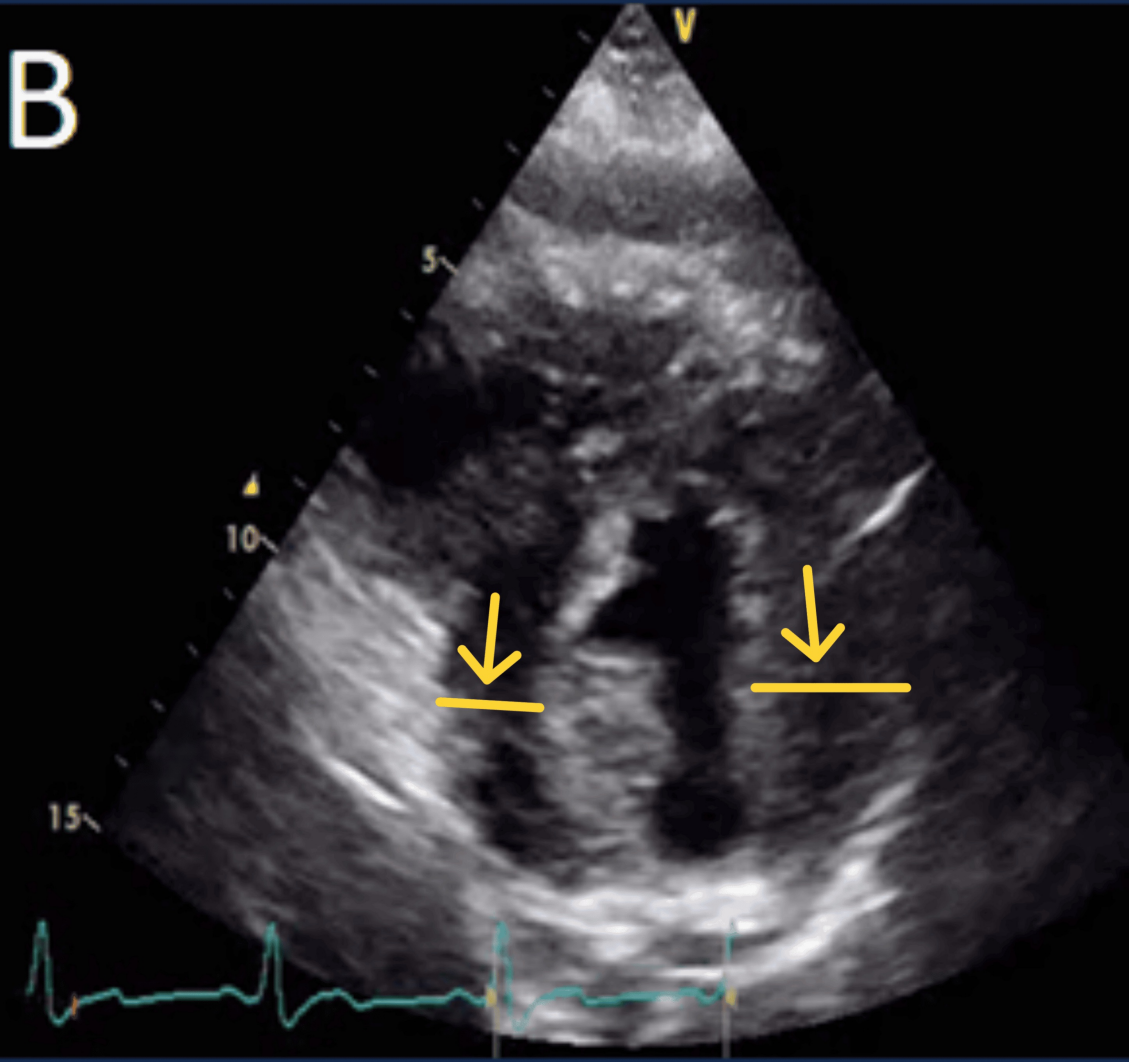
Significant concentric left ventricular hypertrophy is shown by yellow arrows on the parasternal short-axis views on transthoracic echocardiogram.
